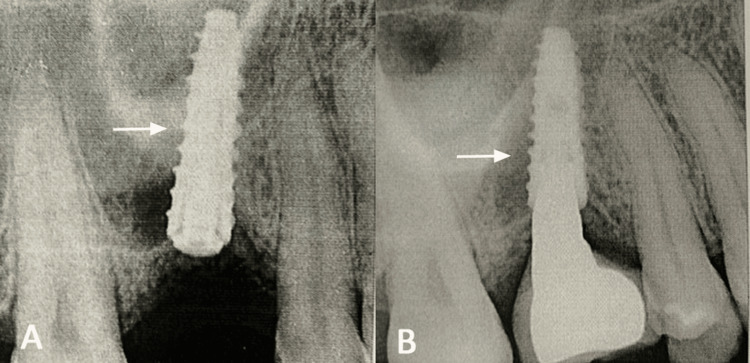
Intraoral periapical radiograph showing the placed dental implant for #26 (A) and at one-year follow-up showing the stable implant (B)
